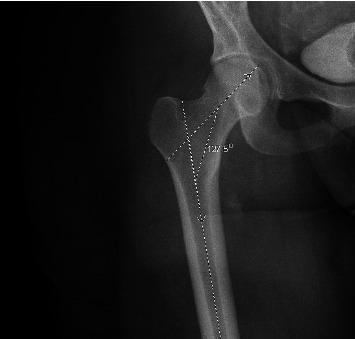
Measurement of CCD angle via x-ray image in EMR/PACS, with 127.5 degrees representing the CCD angle.
